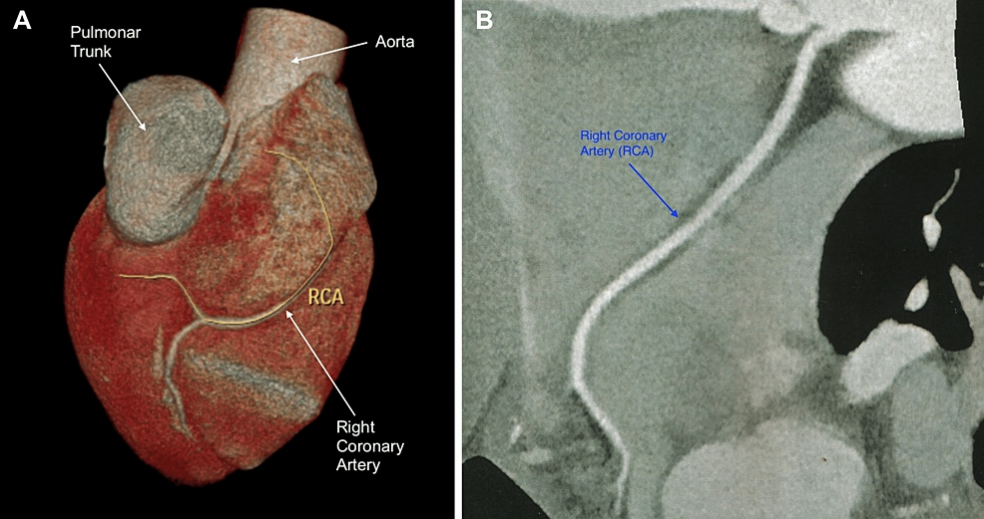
A: Myocardial computed tomography angiography showing a short course of the right coronary artery, with an ostium between the aorta and the RV outflow tract; B: right coronary artery course RV - right ventricular, RCA - right coronary artery
